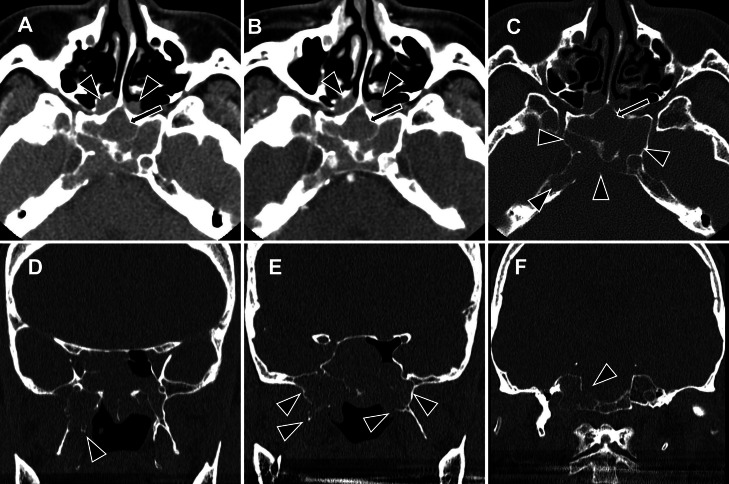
The mass in the sphenoid sinus on CT. (A) Noncontrast axial CT, (B) contrast-enhanced axial CT, (C) axial CT with bone window settings, and (D-F) coronal CT with bone window settings. A large mass is identified originating in the sphenoid sinus (A, B, arrows), with extension into the bilateral posterior ethmoid sinuses and nasal cavity (A, B, arrowheads). The tumor demonstrates heterogeneous enhancement following contrast administration (B). Widespread osteodestruction is evident. The sphenoid bone shows severe involvement, including the posterior aspects of the greater wings (right > left) (C, D, E) and the superior portions of the pterygoid plates (on the right, both plates; on the left, the medial plate only) (D, E). The lesser wings, however, are preserved. The destruction also involves the clivus and posterior occipital bone, with right-sided predominance (C, F).
